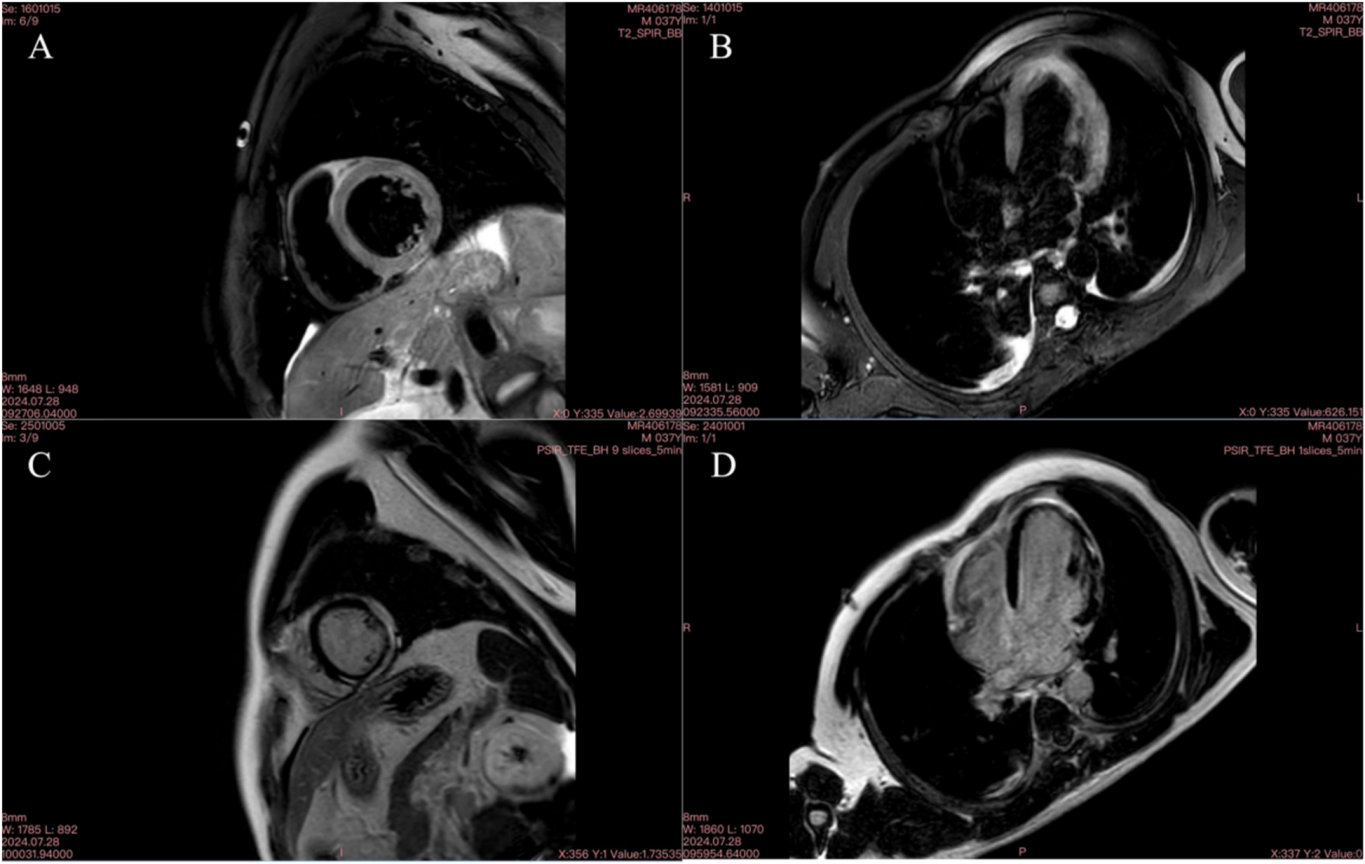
Cardiac MR image on September 28, 2024. (A) T2 fat-suppressed image of the left ventricular short axis showing multiple oedemas; (B) T2 fat-suppressed image of the four-chambered heart showing multiple oedemas; C delayed enhancement of a small amount of the subepicardial myocardium in the lateral wall of the short axis of the left ventricle; D delayed enhancement of a small amount of the subepicardial myocardium in the lateral wall of the four-chambered heart.
